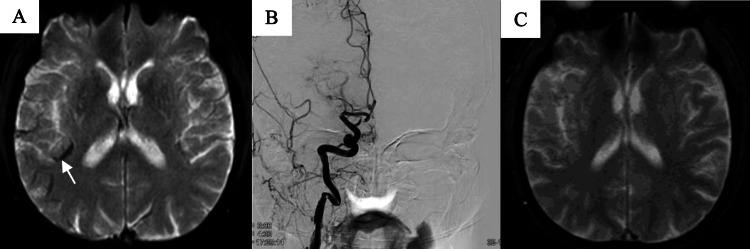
Sample T2* magnetic resonance images showing medullary asymmetrical vein sign A: T2* images of a case with right M1 proximal occlusion. Medullary AVS are visible on the affected side (white arrow). B: No LSAs are visible on the preoperative right ICA injection. C: Postoperative (within 48 hours) T2* images show no medullary AVS. LSAs: lenticulostriate arteries; ICA: internal carotid artery; AVS: asymmetrical vein sign
